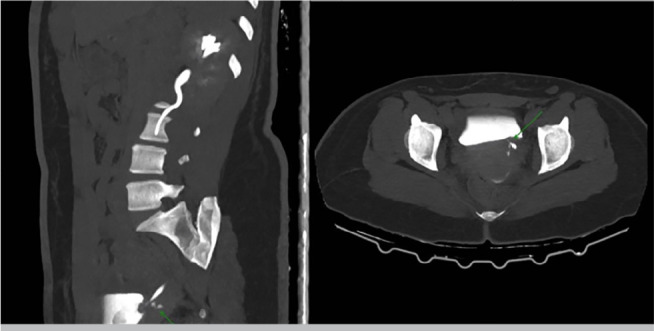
Computed tomography with intravenous contrast demonstrates a fistula
